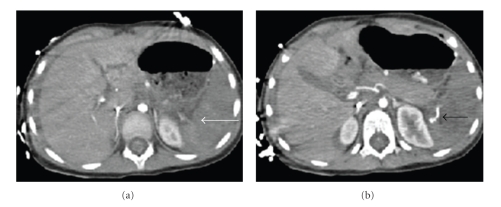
Contrast enhanced CT of the abdomen showing relative hypodensity of the spleen (white arrow), with extravasation of the contrast at the hilum suggestive of active bleeding (black arrow).
